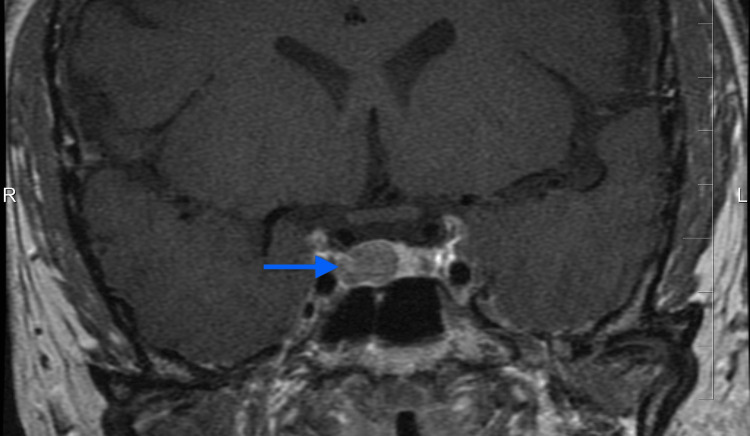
Preoperative pituitary MRI demonstrating a right-sided microadenoma (blue arrow)
